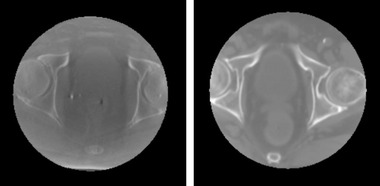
Axial view of a D${}_{r}$ CBCT (left) and a D${}_{s}$ CBCT (right)
